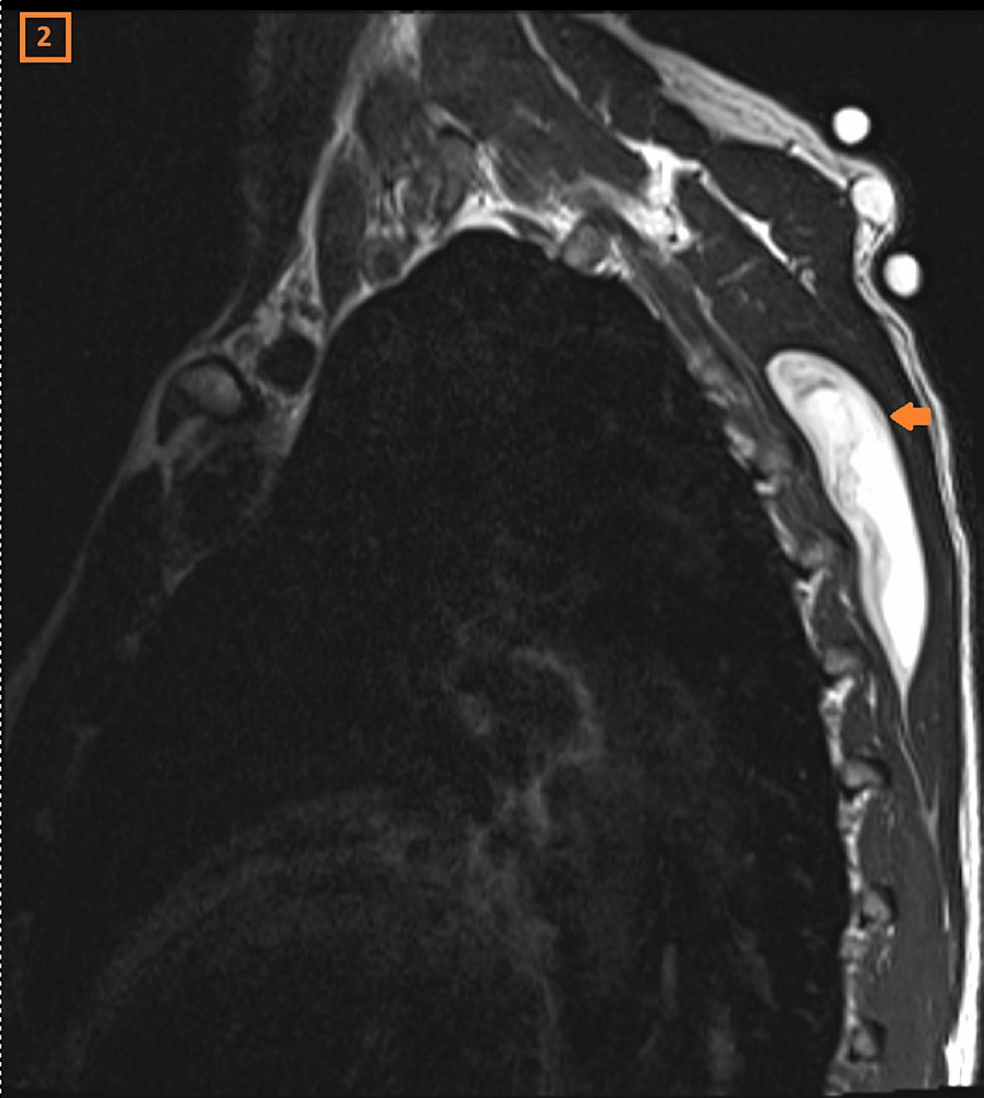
Cystic lesion as seen on sagittal T2-weighted image
